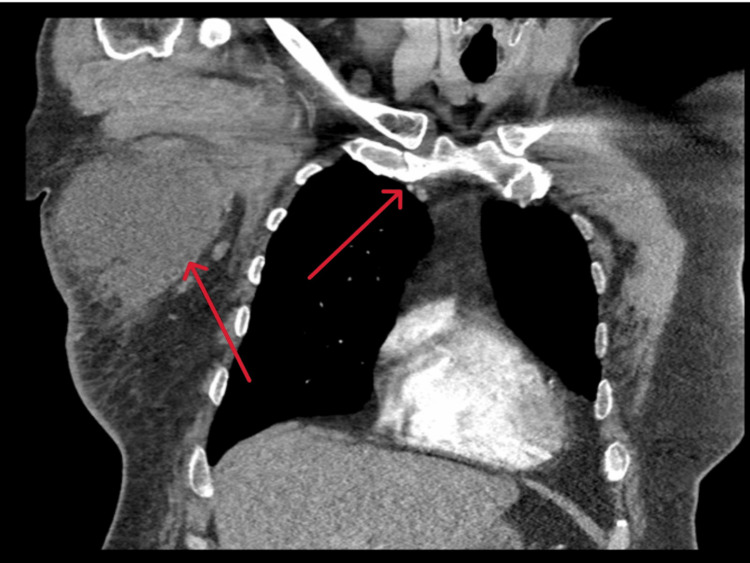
Computed tomography image showing an irregularly shaped peribronchial nodule in the right upper lobe along with the collection in the right axilla and breast
